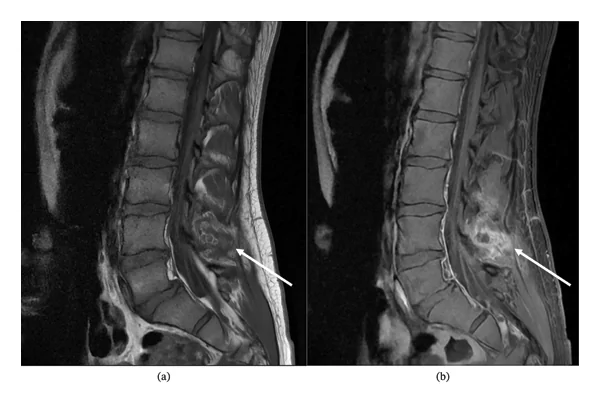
(a) Sagittal MRI of the lumbar region (a gadolinium contrast‐enhanced T1 sequence with fat saturation). The white arrow indicates peripheral enhancement of the bursa, associated with inflammation of the interspinous bursa between the third and fourth lumbar vertebrae (L3 and L4). There was no disc or bone involvement. (b) Sagittal MRI of the lumbar region (a gadolinium contrast‐enhanced T1 sequence with fat saturation). The white arrow indicates peripheral enhancement of the bursa, associated with inflammation of the interspinous bursa between the third and fourth lumbar vertebrae (L3 and L4). There was no disc or bone involvement.
